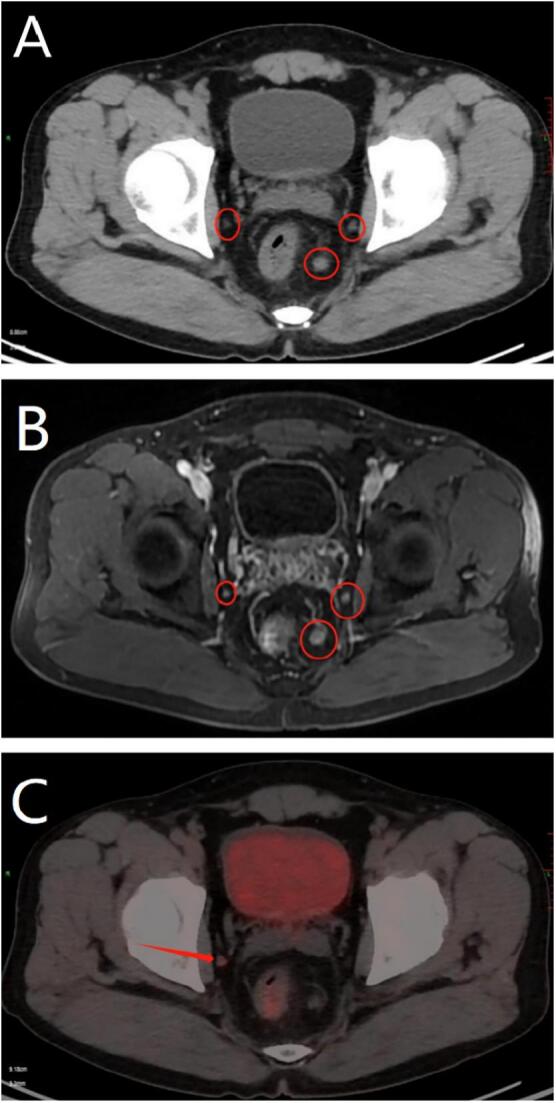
CT(Fig. A) and MRI(Fig. B) indicated many enlarged lymph nodes in the mesorectum and on the both sides of the lateral pelvic space(red circle). PET-CT(Fig. C) showed that the lymph nodes in the mesorectum was positive. (For interpretation of the references to colour in this figure legend, the reader is referred to the web version of this article.)
